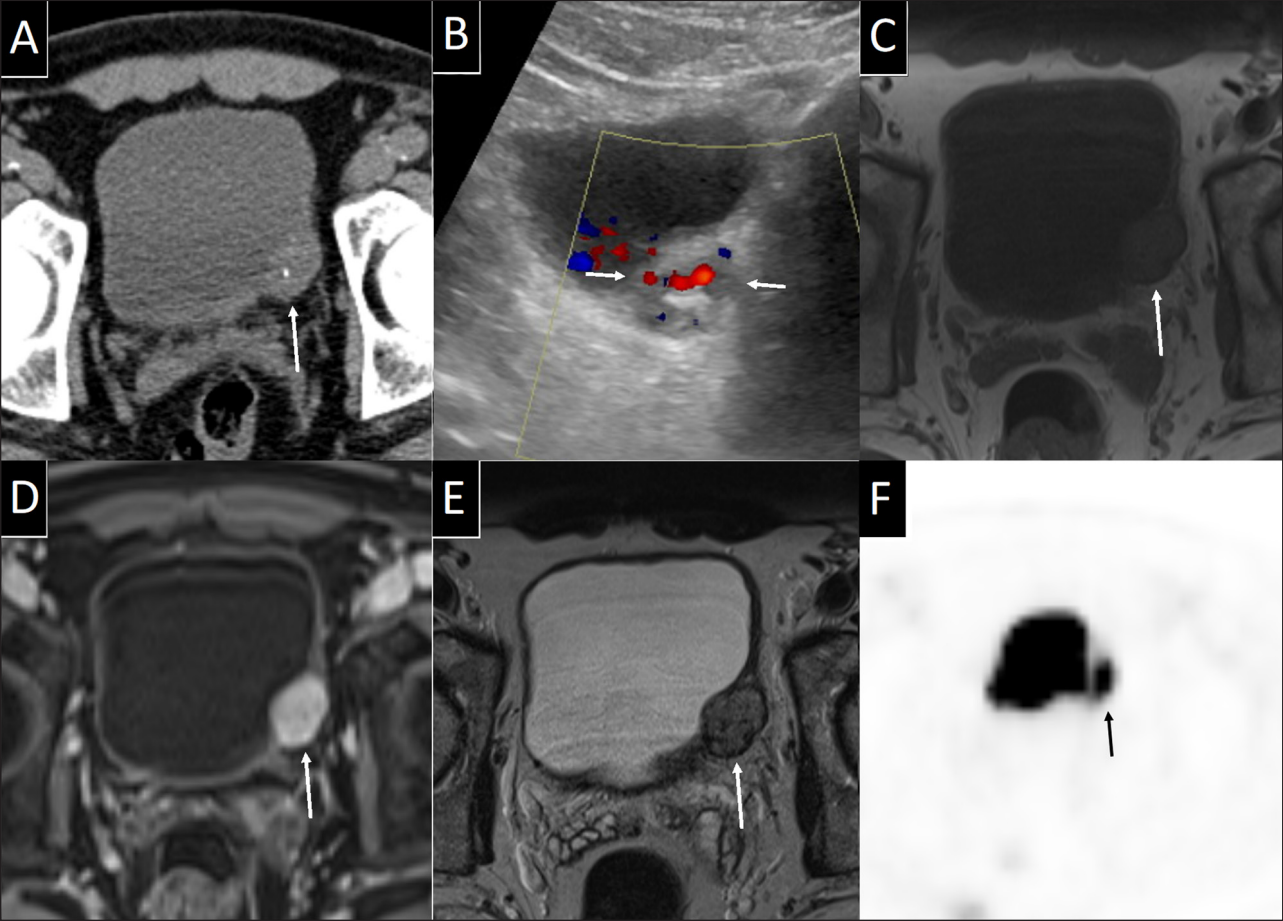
(A) Axial non-contrast enhanced CT image showing the incidental ovoid soft tissue mass (white arrow) with a punctate calcification in the left posterolateral bladder wall. (B) Doppler ultrasound image showing the lesion (arrows) to have a heterogeneous echogenicity, and marked Doppler signal revealing high vascularity. (C) Axial T1-weighted MRI shows the lesion (arrow) to have an intermediate T1 signal and (D) axial fat suppressed T1 after i.v. gadolinium shows marked and homogeneous lesion contrast enhancement (arrow). (E) Axial T2 showing the lesion (arrow) to be located intramurally, with intact overlying urothelium. and having a heterogeneous moderately high T2 signal. (F) Axial F-18 FDG PET image shows moderate FDG uptake of the lesion (arrow). Also note the renally excreted FDG in the bladder lumen.
